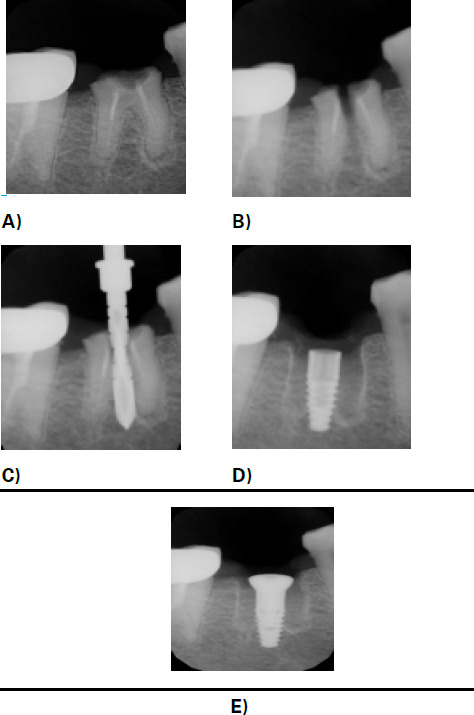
Radiographic image showing A) remaining roots with under obturated canals with no coronal portion, B) separation of mesial and distal roots till furcation, C) achieving center point after initial osteotomy in between mesial and distal roots, D) placement of implant in exact point after roots removal, E) radiograph showing gingival former placement.
